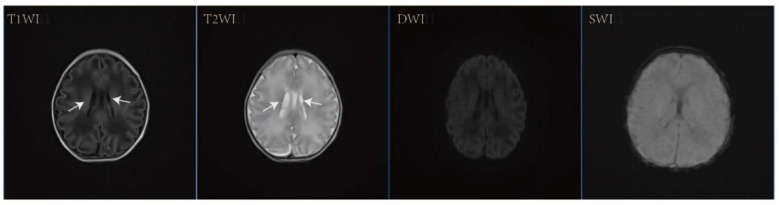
MRI images of subependymal cyst located in the body of the lateral ventricle.
